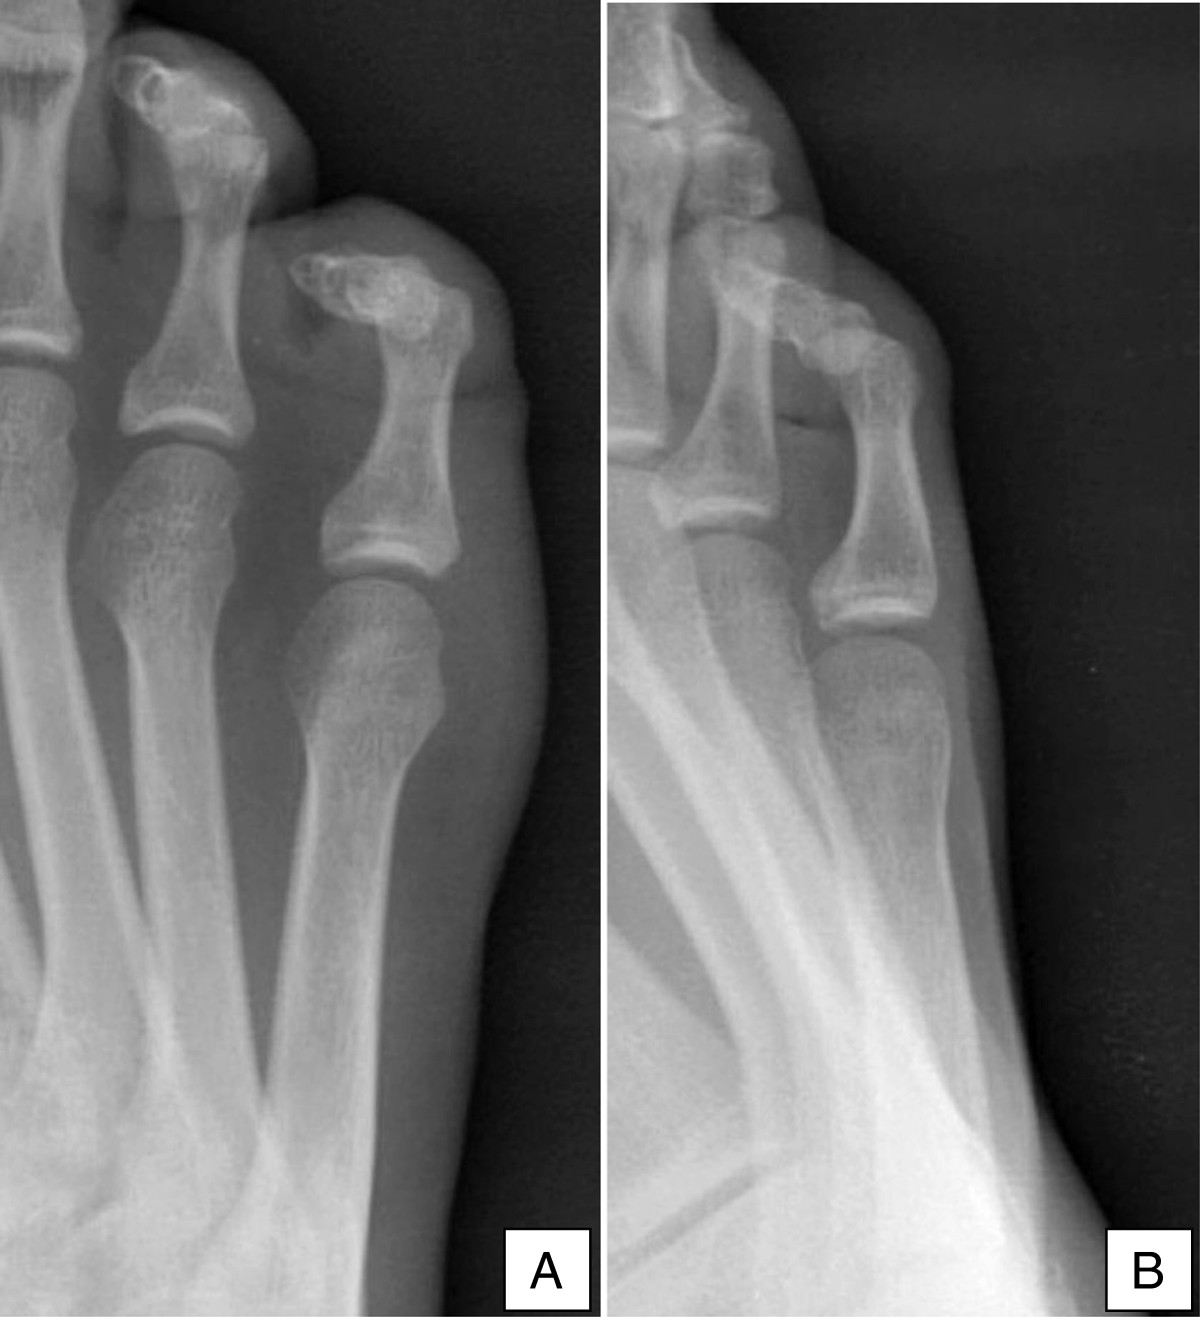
Radiograph showing number of phalanges. (A) Dorsoplantar radiograph showing inconclusive number of phalanges (B) Internal oblique radiograph of the same foot conclusively showing that the fifth toe is bifalangeal.
